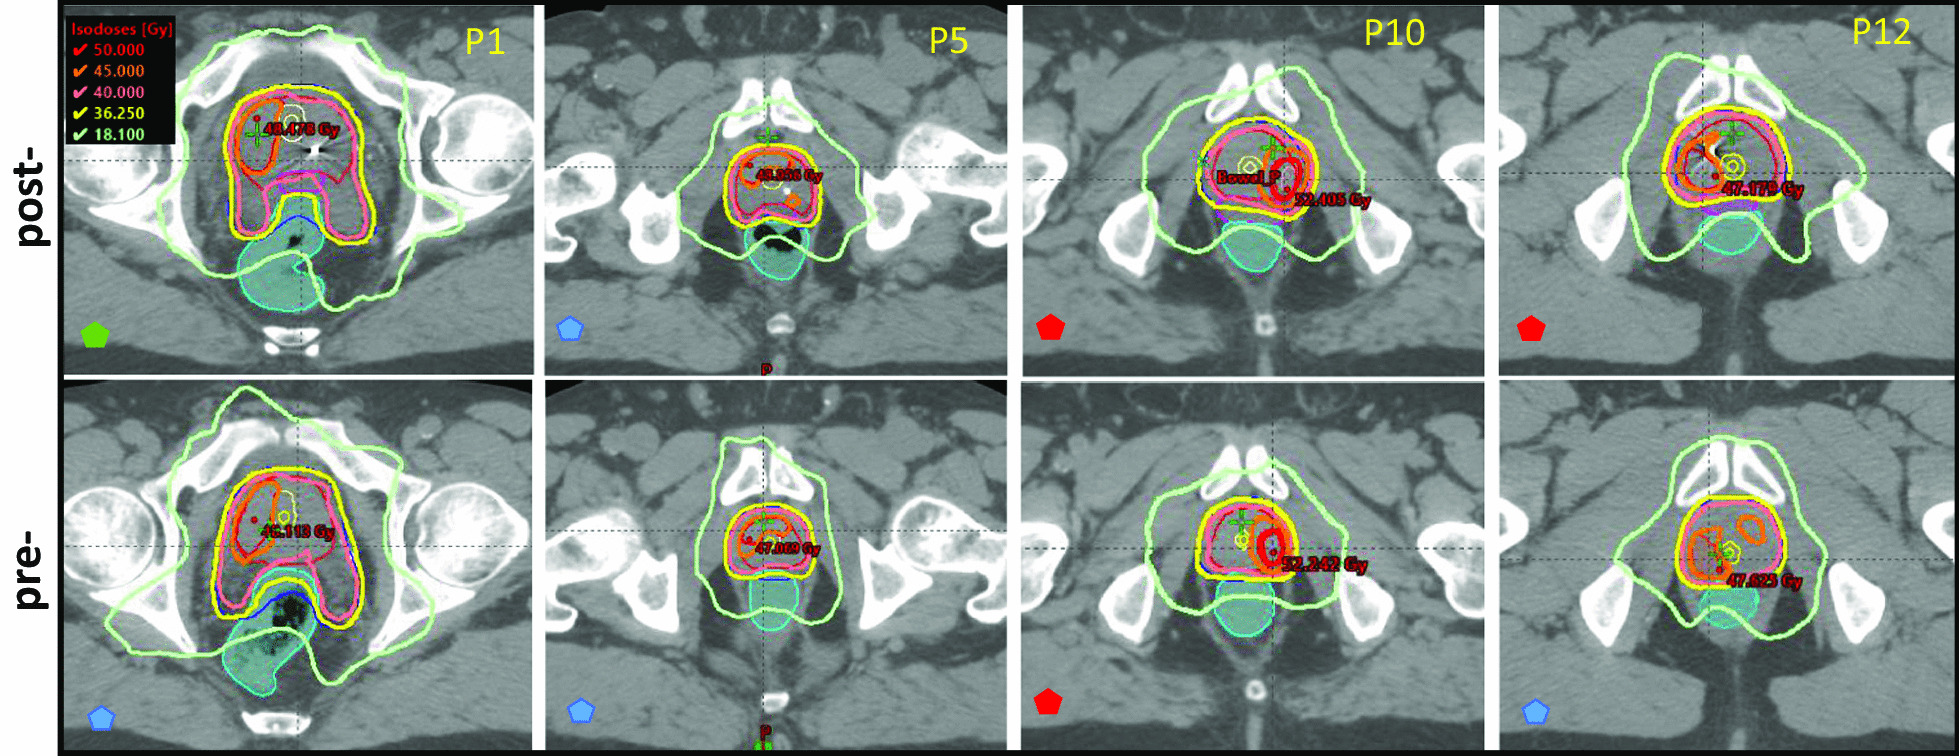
Example axial views of pre- and post-spacer CT scans and isodose levels for matched dose plans. The prescribed dose to the prostate Tpsv (shown in red) was 40 Gy, the PTVpsv (blue) was 36.25 Gy and the CTVpb (outlined in red). The coloured geometrical shapes (pentagons) on the lower left side of the figures indicate achievable DIL dose level; 50 Gy (red), 47.5 Gy (green) or 45 Gy (blue). Urethra and urethra_PRV are outlined yellow, rectum is cyan and the hydrogel spacer in is outlined in magenta colour. The other lines indicate selected iso-dose lines as indicated by the key
